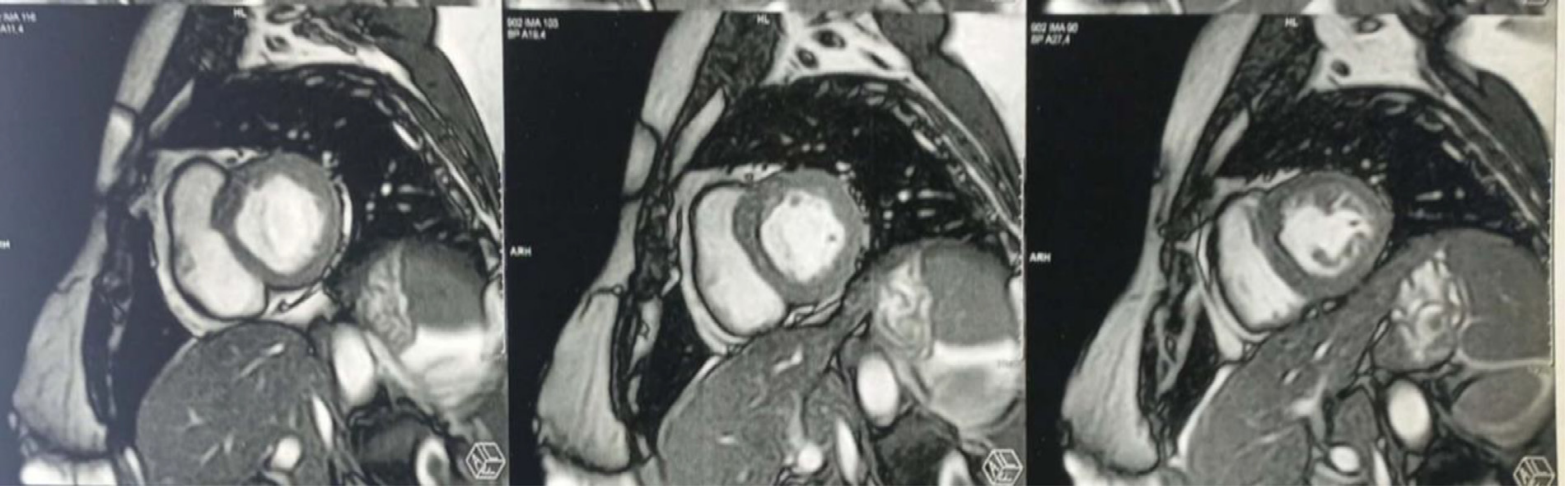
T2 short-axis cardiac MRI sequence demonstrating significant edema in the lateral wall.
